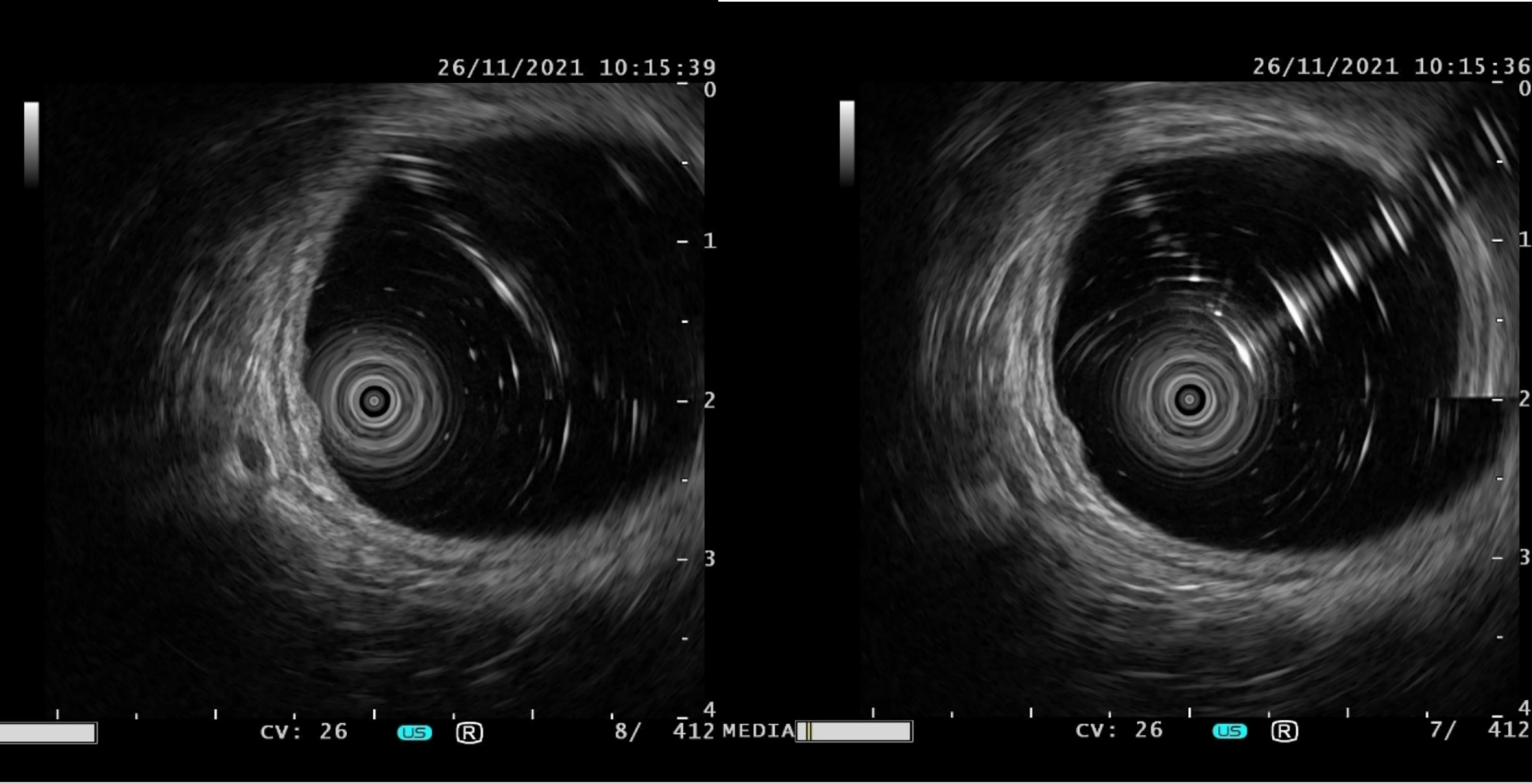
The typical manifestations of lesions under endoscopic ultrasonography were originated from the mucous layer or submucosa, and the internal echo was homogeneous, showing low or moderately low echo, and the boundary was clear.
